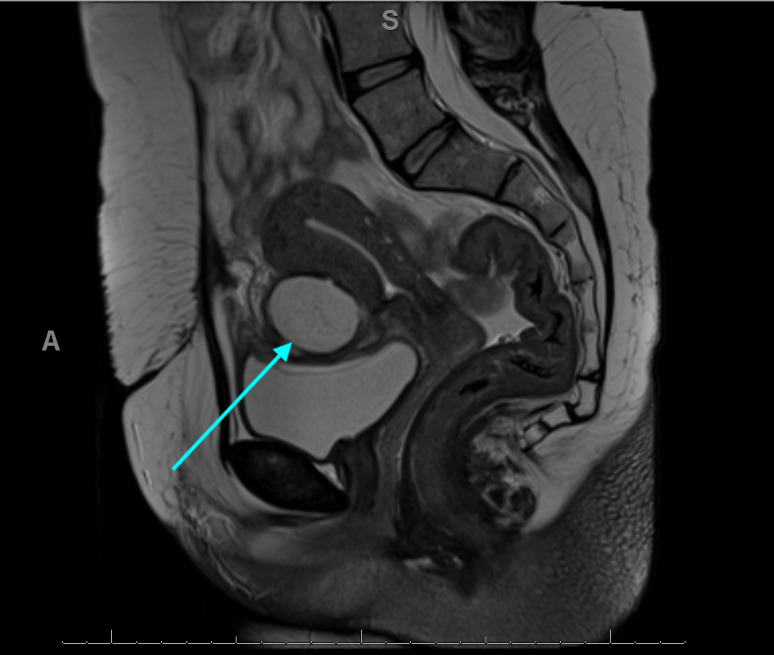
Case 2 Sagittal section of MRI demonstrating a fluid collection at the uterine vesicular fold closely related to the caesarean section scar.
